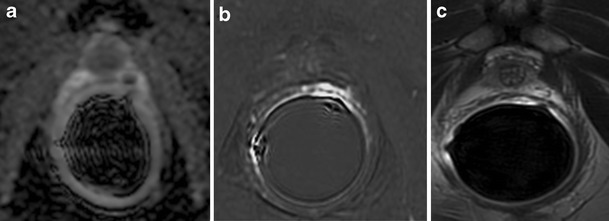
Mispositioned endorectal coil. a ADC map (DWI acquired with b value = 1000 mm^2^/sec and b 3000 mm^2^/sec) showing focal low signal intensity on the left side of the apex. b Gradient-echo T1-weighted subtracted image showing at the same site strong focal enhancement. According to the PIRADS v2 scoring system, the score for DWI and DCE is, respectively, 4 and +, which means highly clinically significant cancer is likely to be present. c Axial T2-weighted fast spin-echo image (6006/110) display no differences in signal intensity between left and right postero-lateral aspect, and it represents the decisive sequence confirming the mispositioned endorectal coil
